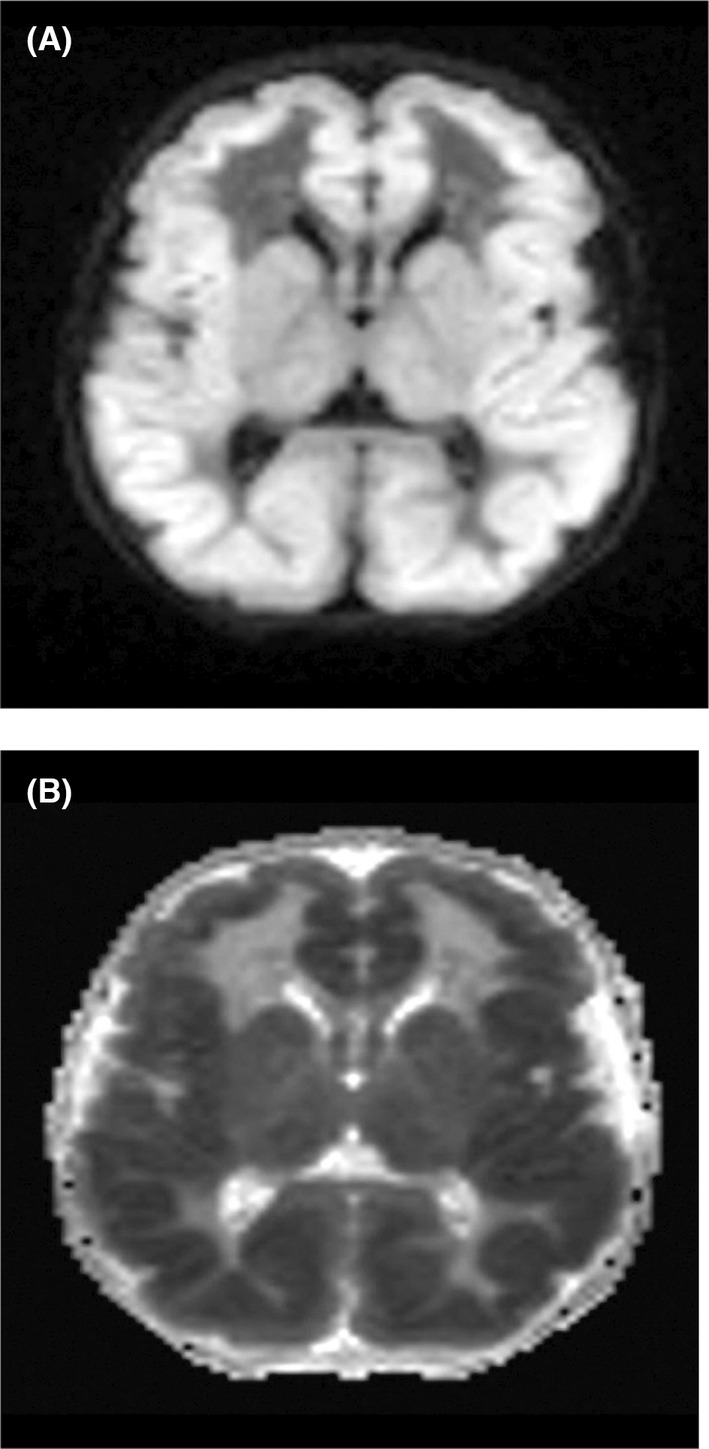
Brain MRI. Axial diffusion‐weighted imaging (DWI), (A) apparent diffusion coefficient (ADC) image. (B) Diffusion restriction (of bright signal in DWI and low signal in ADC images).
